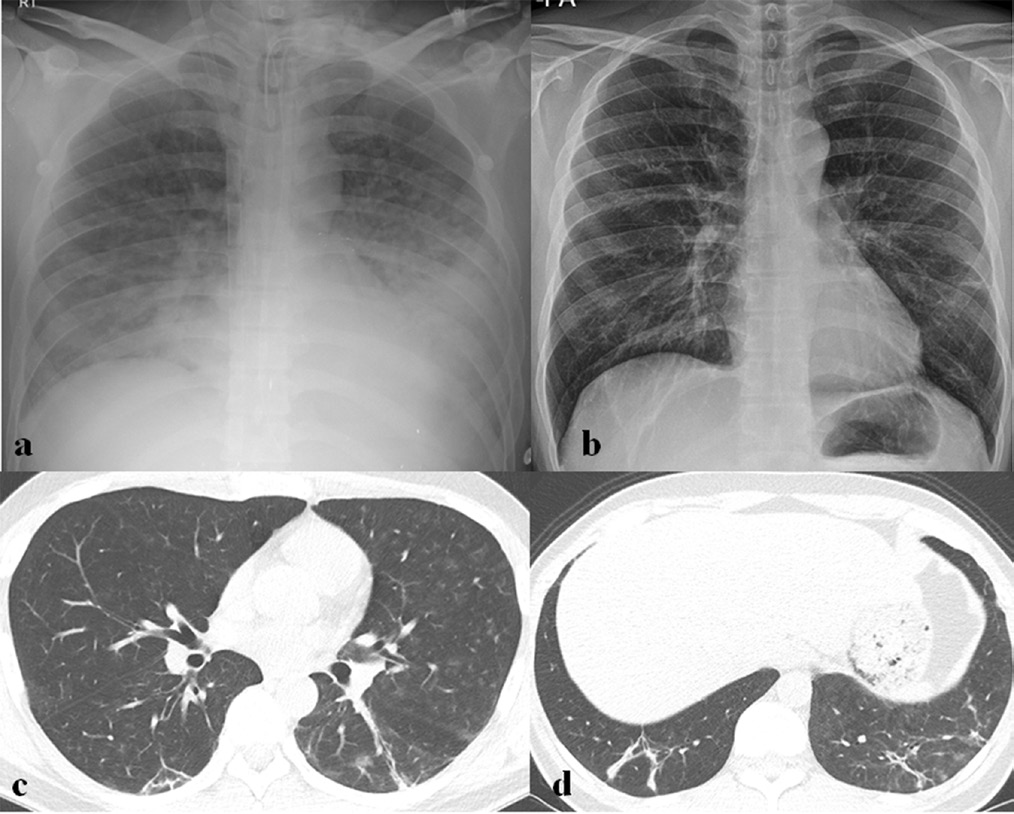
A 32-year-old male with MERS-CoV infection. (a) Chest radiograph (CXR) obtained on 15 days after symptom onset shows consolidation and ground-glass opacities in both middle to lower lung zones. (b) Follow-up CXR obtained 50 days after symptom onset shows linear and patchy opacities in both lower lung zones, which may represent fibrosing sequelae. (c, d) Follow-up chest CT scans obtained on the same day with (b) show parenchymal bands, linear opacities, and traction bronchiectasis in both lower lobes, suggesting remnant fibrosis.
